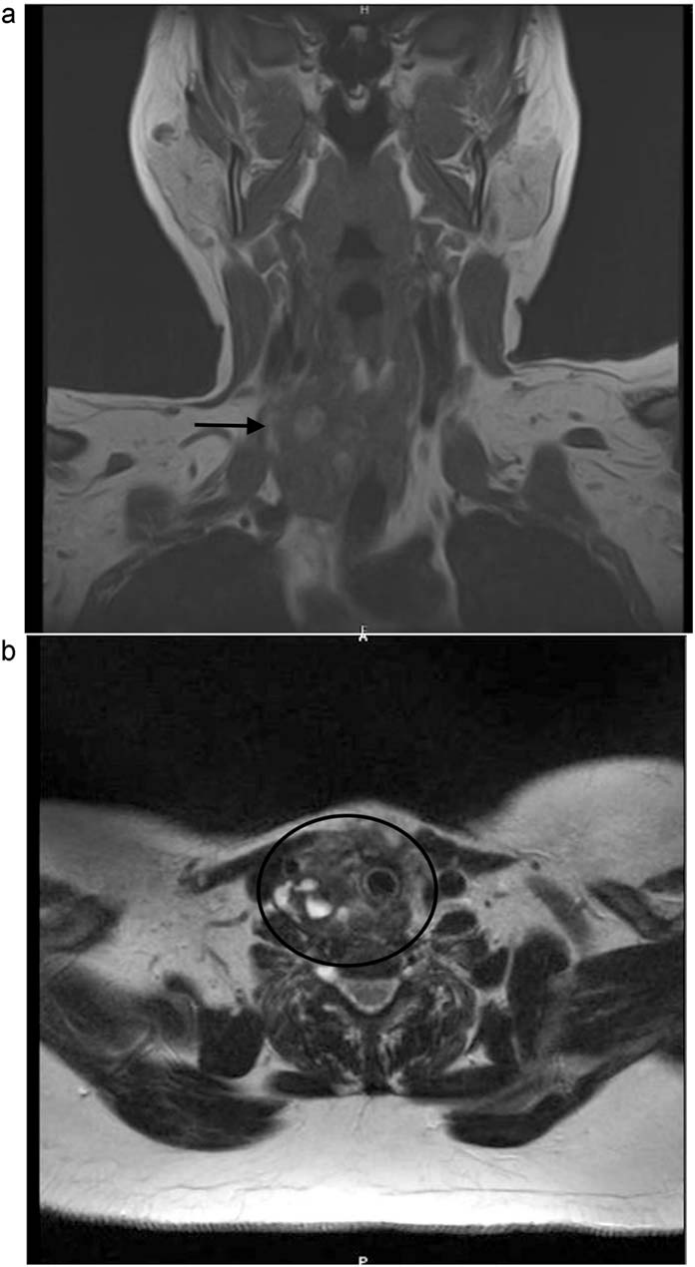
(a, b) Neck MRI, showing a mass 3 × 3.2 × 4.8 cm at the right thyroidectomy bed.
